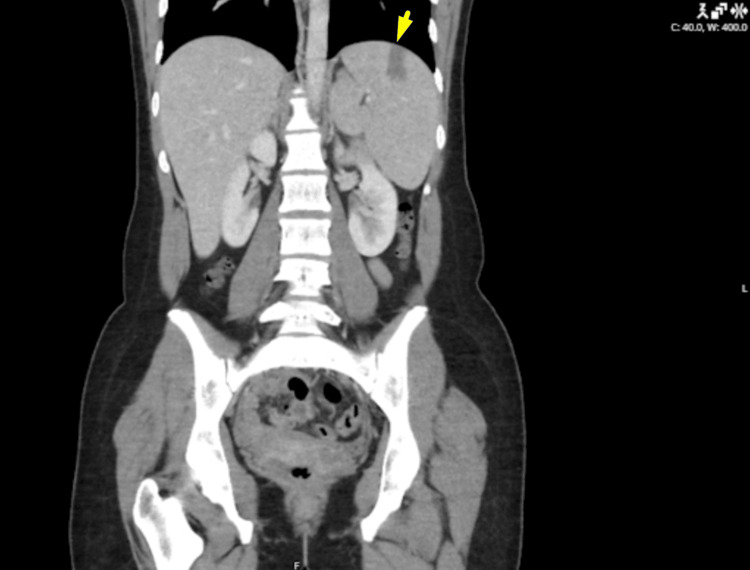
Coronal CT image of the abdomen Splenic infarct visualised on coronal view (indicated by arrow).
